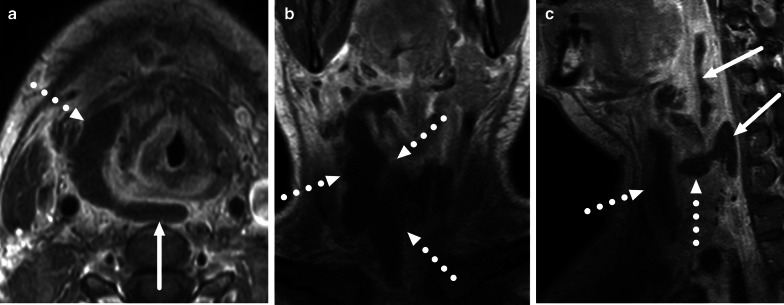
Multi-space abscess on axial (a), coronal (b), and sagittal (c) post-contrast T1-weighted images. A pharyngotonsillar infection was complicated by a large abscess affecting the retropharyngeal (arrows) and visceral and anterior cervical (dotted arrows) spaces
